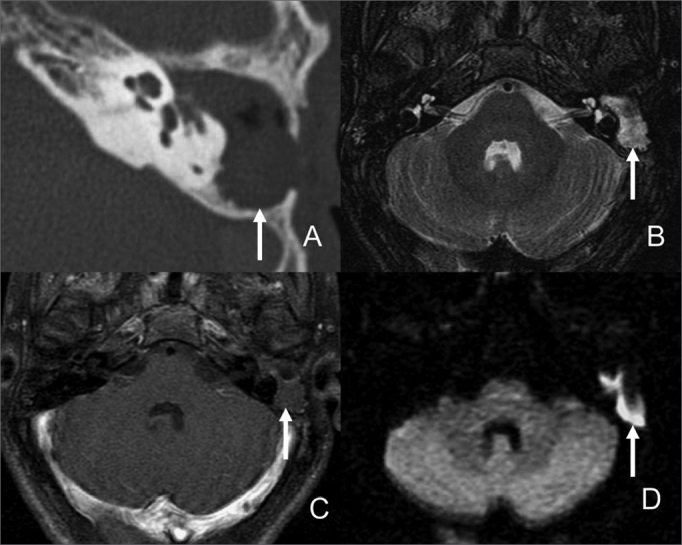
Male patient, 57, with relapsing cholesteatoma in the left mastoid. A- CT scan axial view with unspecific soft tissue in the surgical cavity. B- Axial view on T2; C- Delayed post-contrast axial view on T1; D- Axial view on diffusion imaging. Cholesteatoma resonance images are characterized by hypersignal in diffusion MRI and absence of intravenous contrast uptake.
